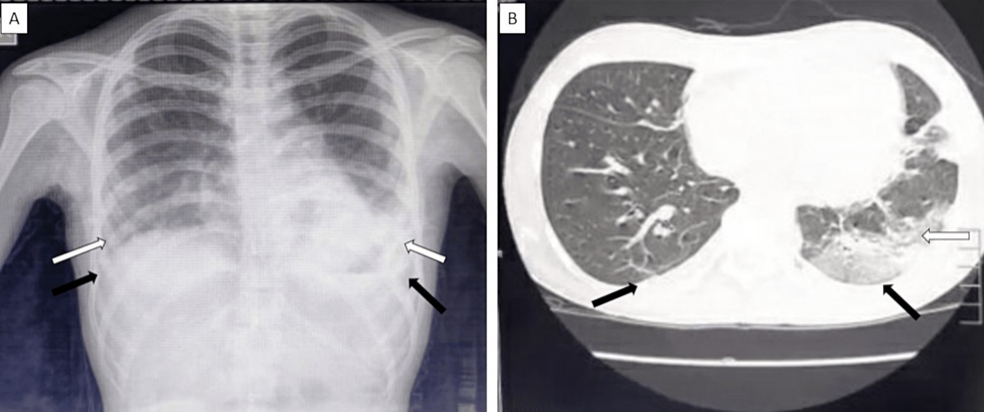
Chest X ray and CT thorax images showing bilateral lower lobe consolidation (white arrows) and pleural effusion (black arrows)
